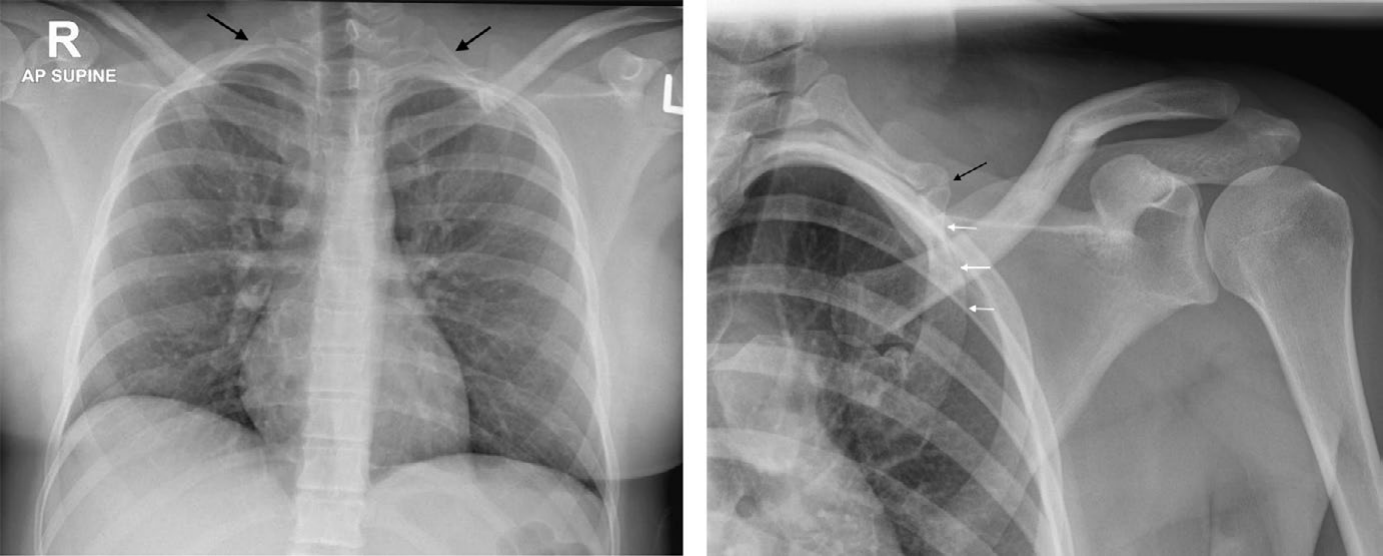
Left (A) AP chest radiograph shows bilateral cervical ribs. Right (B) AP radiograph of the left shoulder shows pseudoarthrosis (black arrow) of the cervical rib with the deformed first rib (white arrows)
